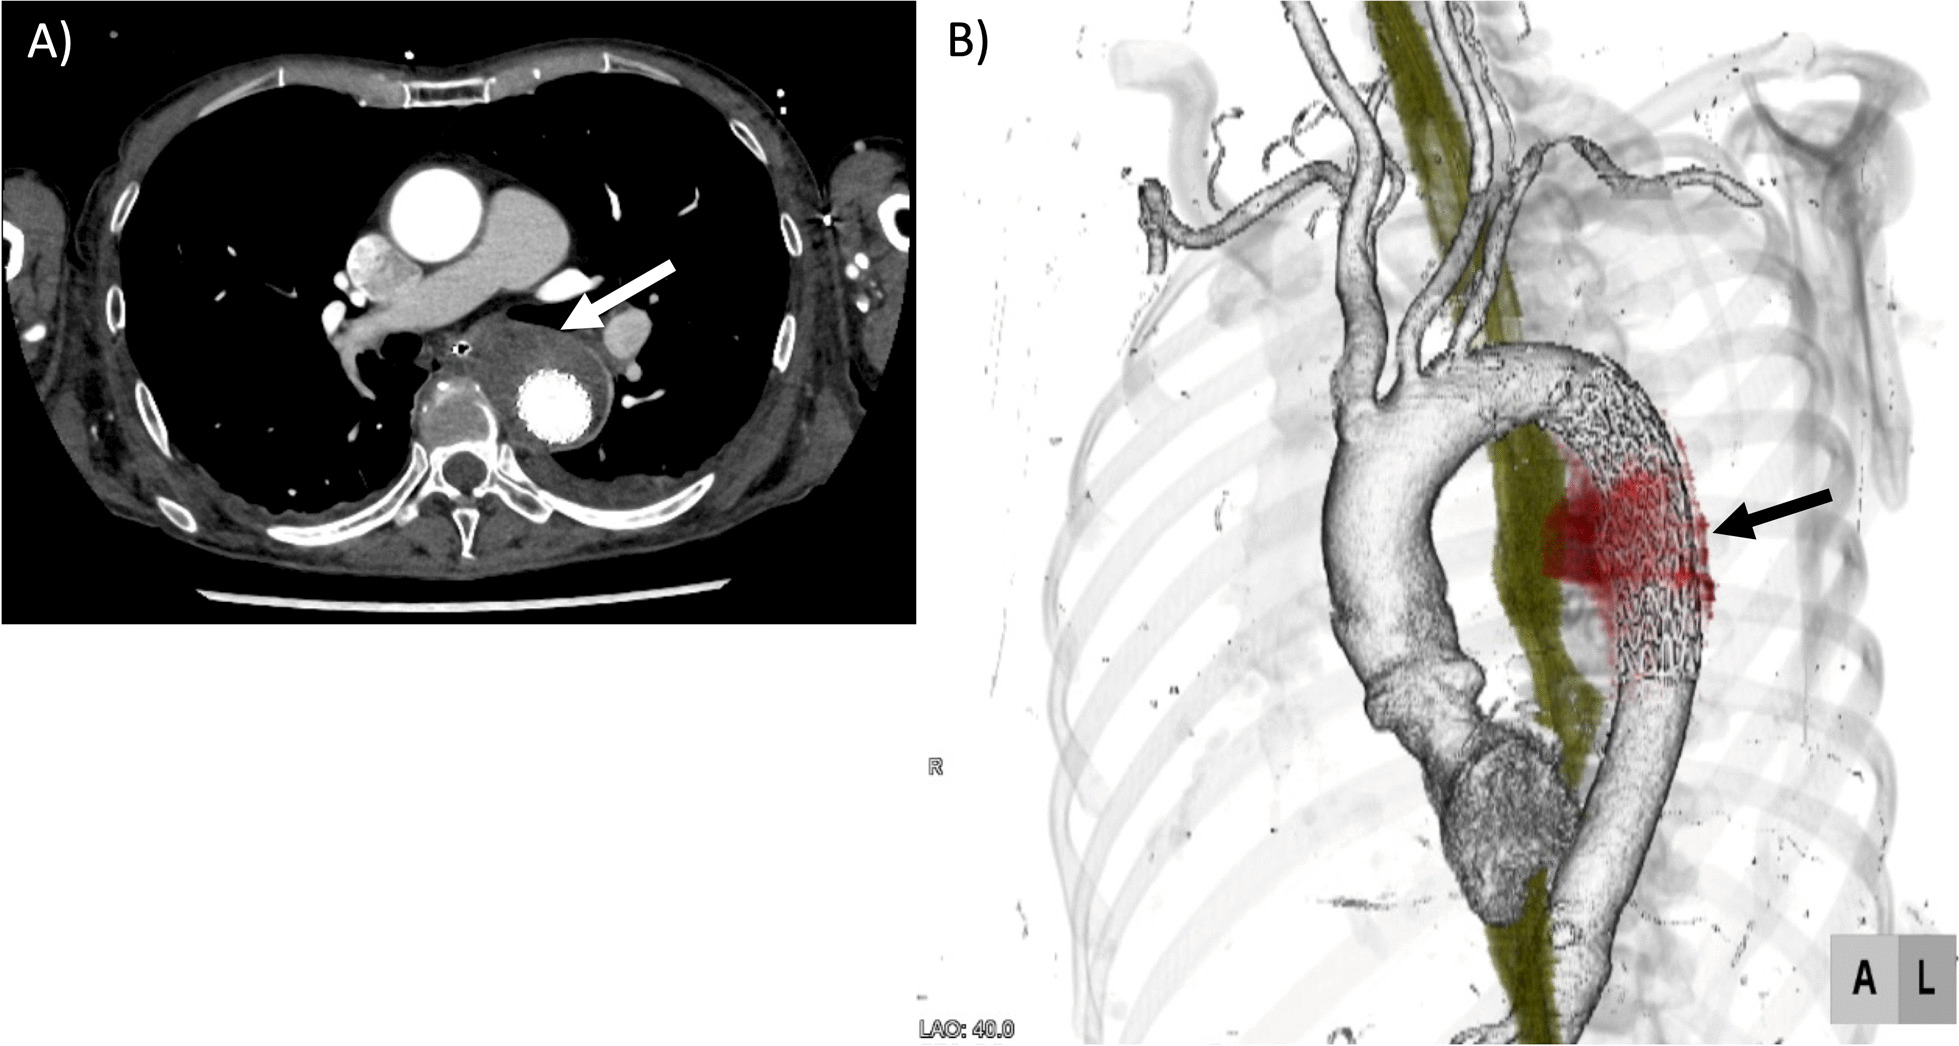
Computed tomography images after thoracic endovascular aortic repair (1 week after surgery). a An axial image after thoracic endovascular aortic repair. This shows that the aneurysm is thrombosed and there is no fistula formation (arrow). b A three-dimensional computed tomography image after thoracic endovascular aortic repair. The saccular descending aortic aneurysm is thrombosed. This shows that the stent-graft is deployed, and the aneurysm is thrombosed (arrow)
